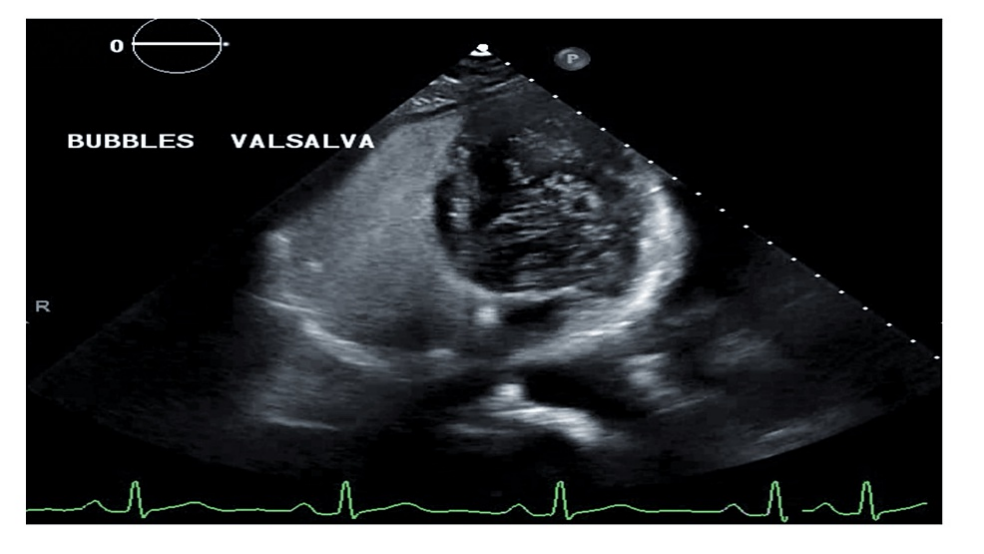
Echocardiogram done showing a positive bubble study on Valsalva suggestive of a patent foramen ovale
